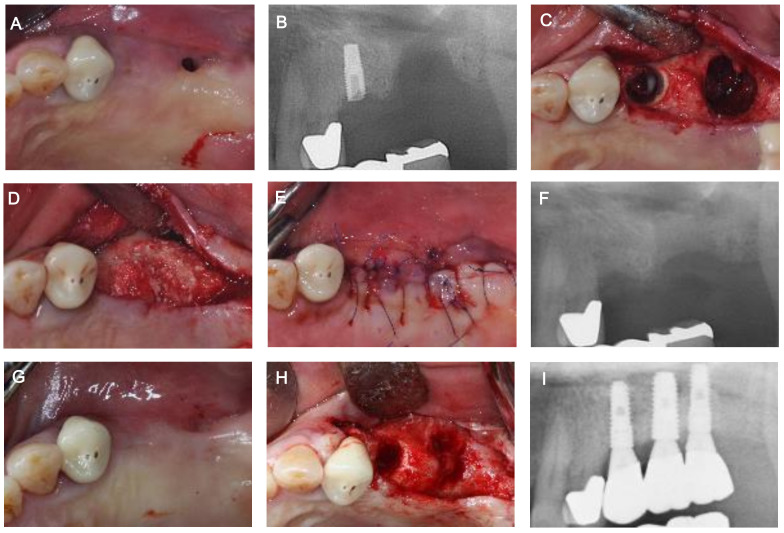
Another case of implant placement after oro-antral fistula (OAF) and oro-antral communication (OAC) closure using an absorbable collagen membrane in the form of a pouch. (A) Pre-operative clinical view of #27. (B) Pre-operative radiograph of #27. (C) Maxillary sinus membrane perforation and an OAC (≥10 mm in diameter). (D) Sinus bone graft with allogenic bone material after collagen membrane application in the sinus by making a pouch shape. (E) Suturing. (F) Post-operative radiograph of #27. (G) Healing condition at 2 weeks post-operatively. The OAF was treated. (H) Clinical view before first-stage implant surgery. Promising hard tissue development at the site of the OAC was observed. (I) Radiograph after prosthesis.
